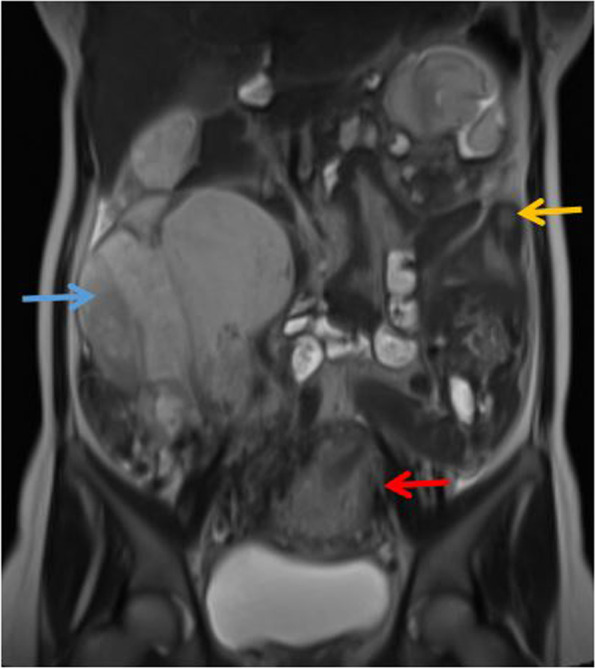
MRI showed an abdominal pregnancy, Placenta (blue arrow), fetus (yellow arrow), and uterus (red arrow)
